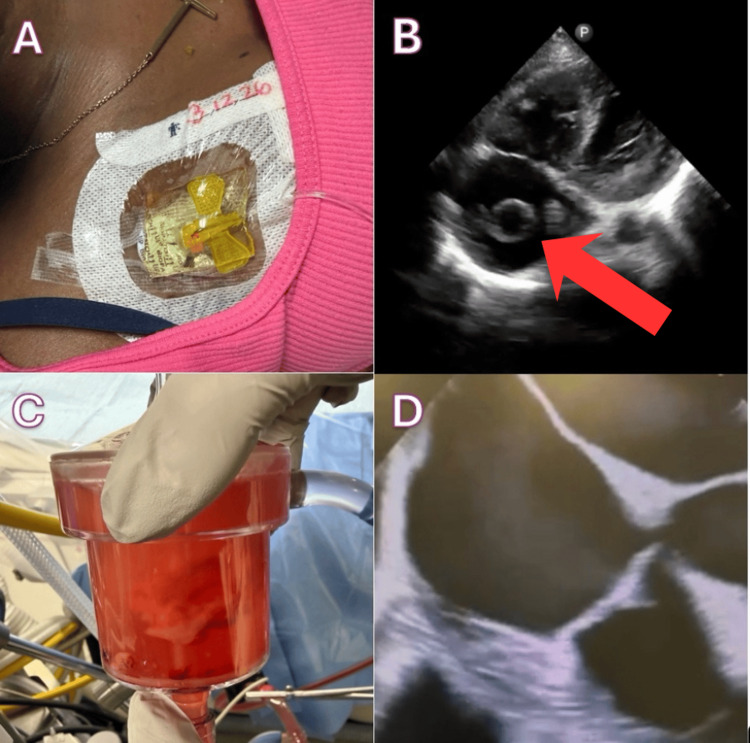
(A) Implanted venous access port present on right chest. (B) Right atrial mass on four apical chamber view, present on bedside POCUS, and later found to measure 3.1×2.2 cm on formal transthoracic echocardiography. (C) Thrombotic material retrieved during AngioVac thrombectomy, demonstrating a large burden of intracardiac thrombus consistent with prior echocardiographic findings of a right atrial mass. (D) Right atrium free of thrombus as shown on intraoperative transesophageal echocardiography. POCUS: Point-of-care ultrasound.
